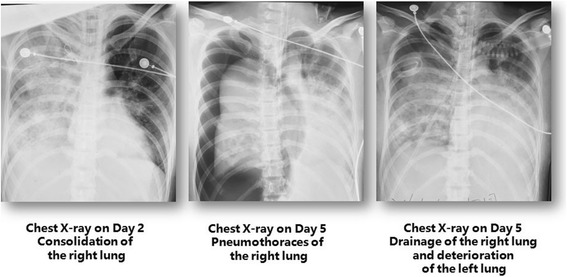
Chest X-rays on day 2 in the outside hospital, on day 5 when right pneumothorax occurred, and on day 5 after a tube was inserted
